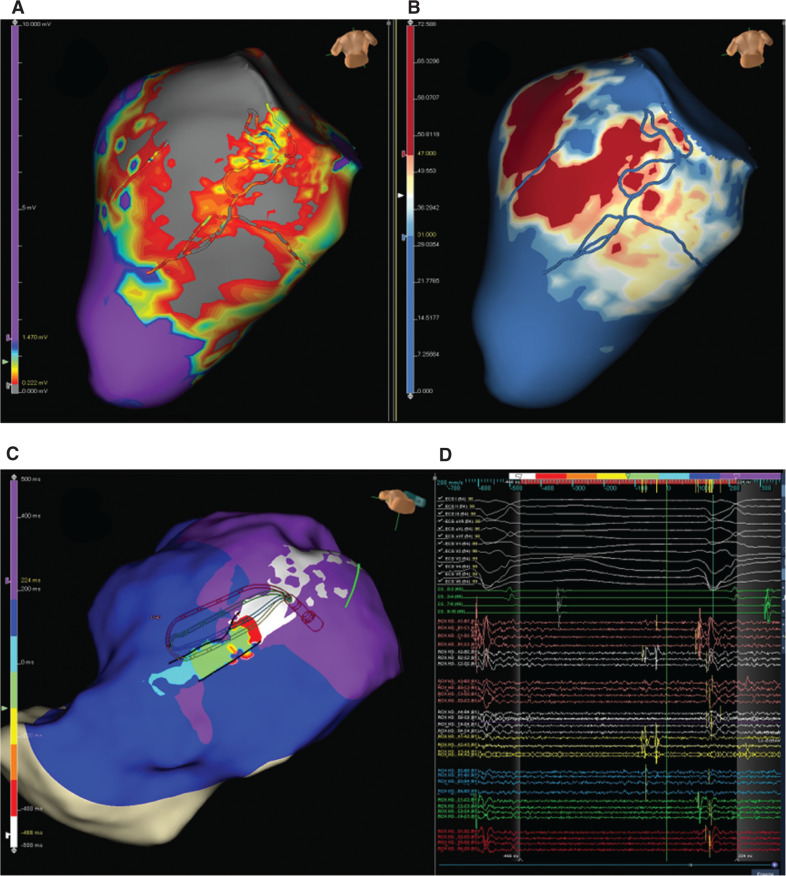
Substrate map in sinus rhythm. A: Two low-voltage channels can be seen in the inferobasal area of the left ventricle. B: ADAS three-dimensional map (ADAS3D Medical, Barcelona, Spain) and the channels visualized with magnetic resonance imaging. C: VT activation map. The VT isthmus is located in the channels seen in A and B. D: Diastolic signals seen in the A spline and the A2–B2 bipole of the HD Grid, located on the isthmus of the VT.
